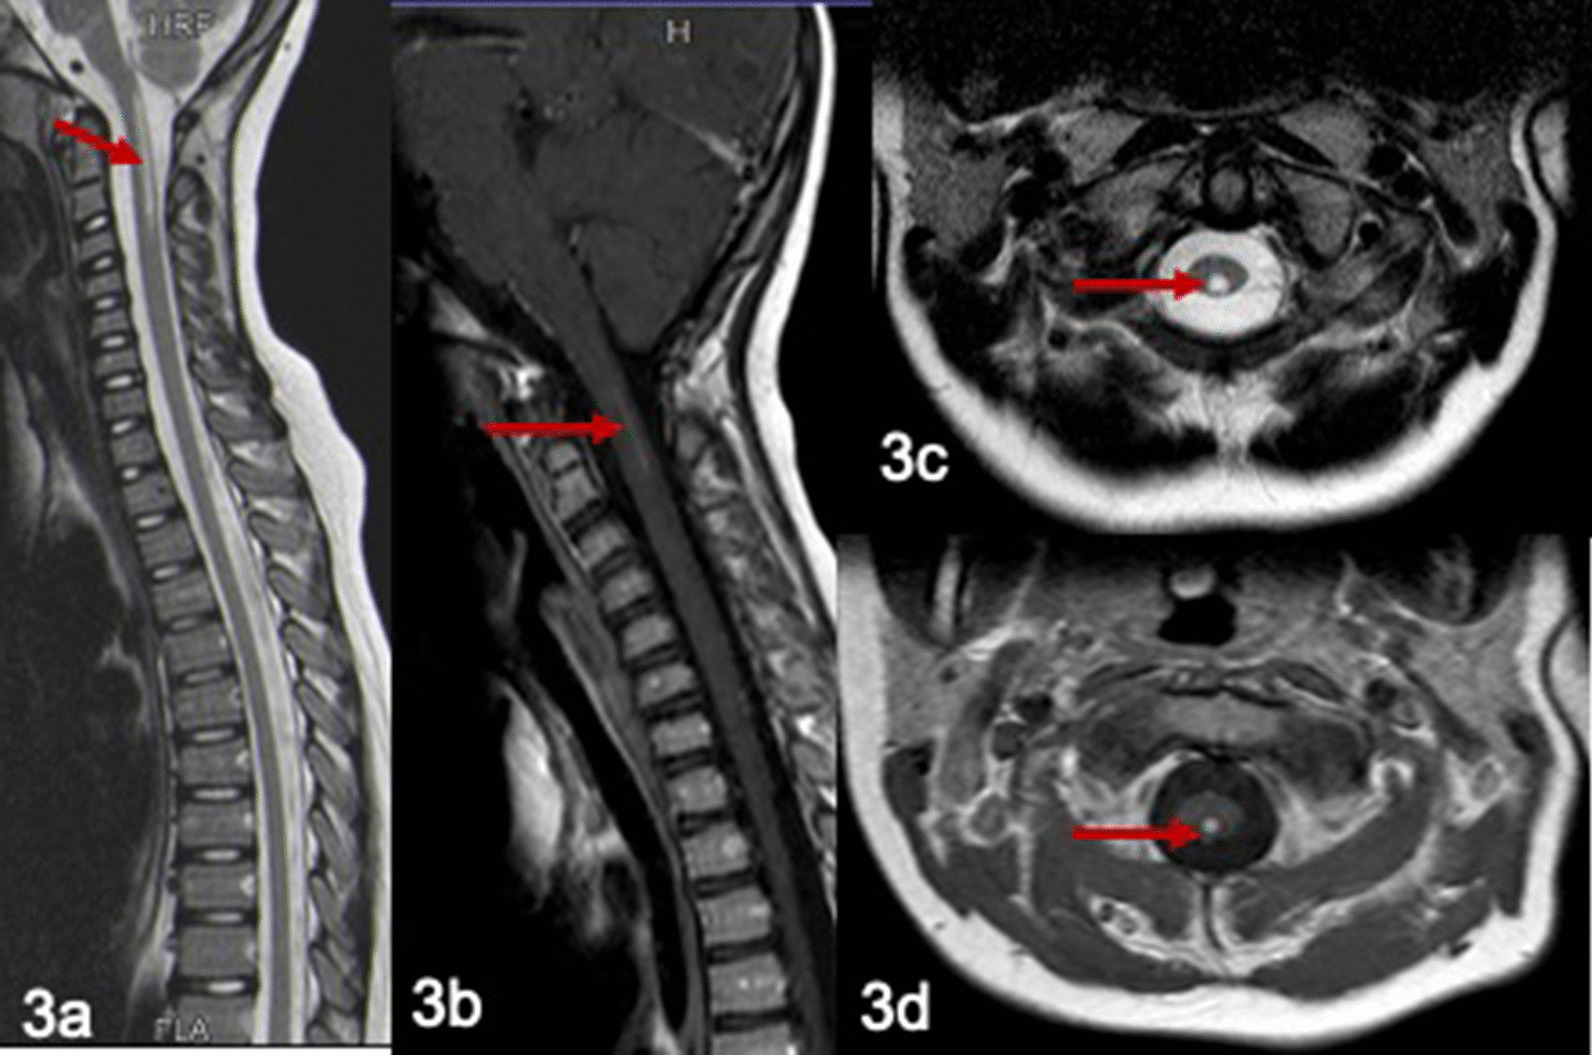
Spinal MRI in patient 2 at 9 years. T2 hyper-intense lesions in the posterior cervical cord extending from the level of the craniocervical junction to the C3 vertebral body (a, sagittal view, c, T2 weighted axial view). The lesions showed enhancement on administration of contrast (b sagittal, d axial view)
